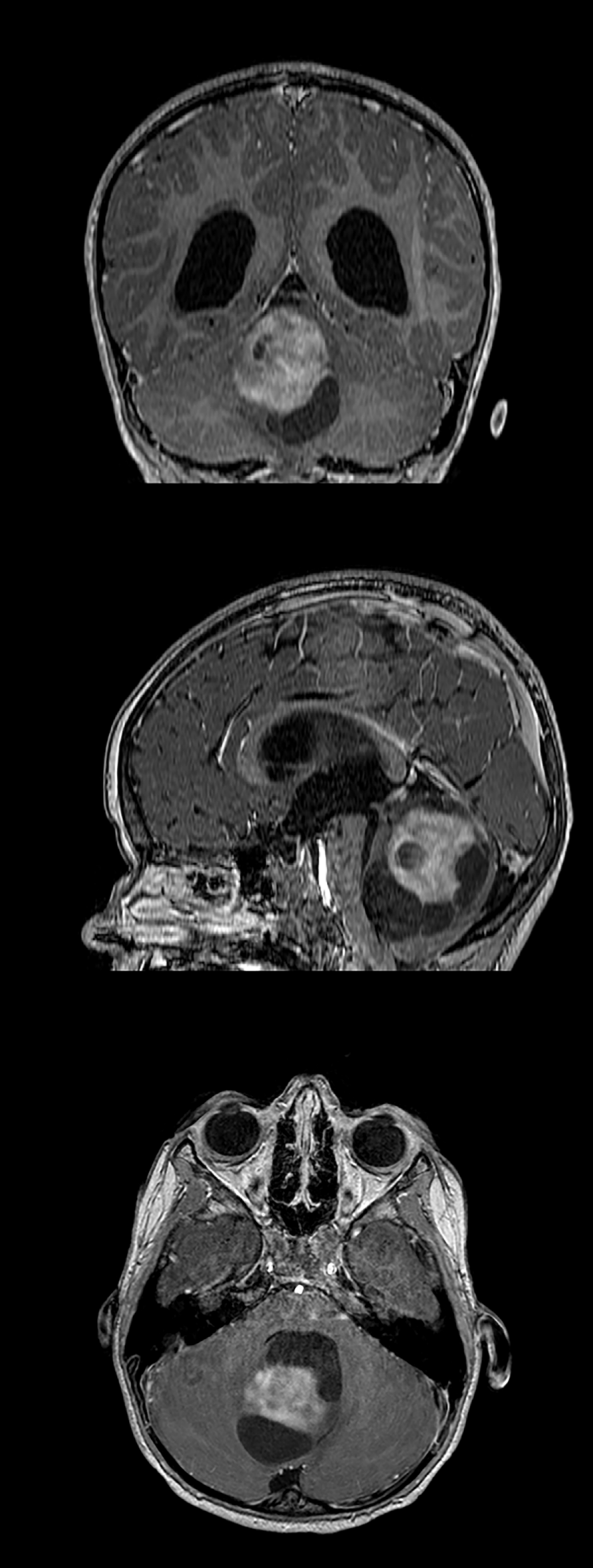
T1-weighted, gadolinium-enhanced coronal, sagittal, and axial cerebral MRI showing a large vermian mass with well-defined margins of approximately 57 × 50 × 44 mm. The mixed structure with a solid, gadolinium-enhancing nodule and multi-lobed cystic component was consistent with the diagnosis of pilocytic astrocytoma. Triventricular hydrocephalus and initial descent of the cerebellar tonsils are also evident
